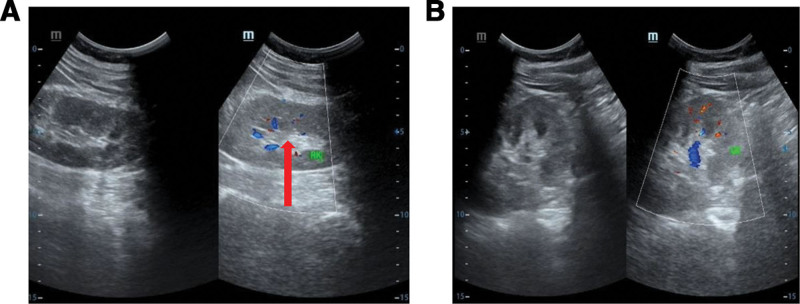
Surveillance ultrasound within 2 months postoperatively following complete resection of a right-sided retroperitoneal tumor.
